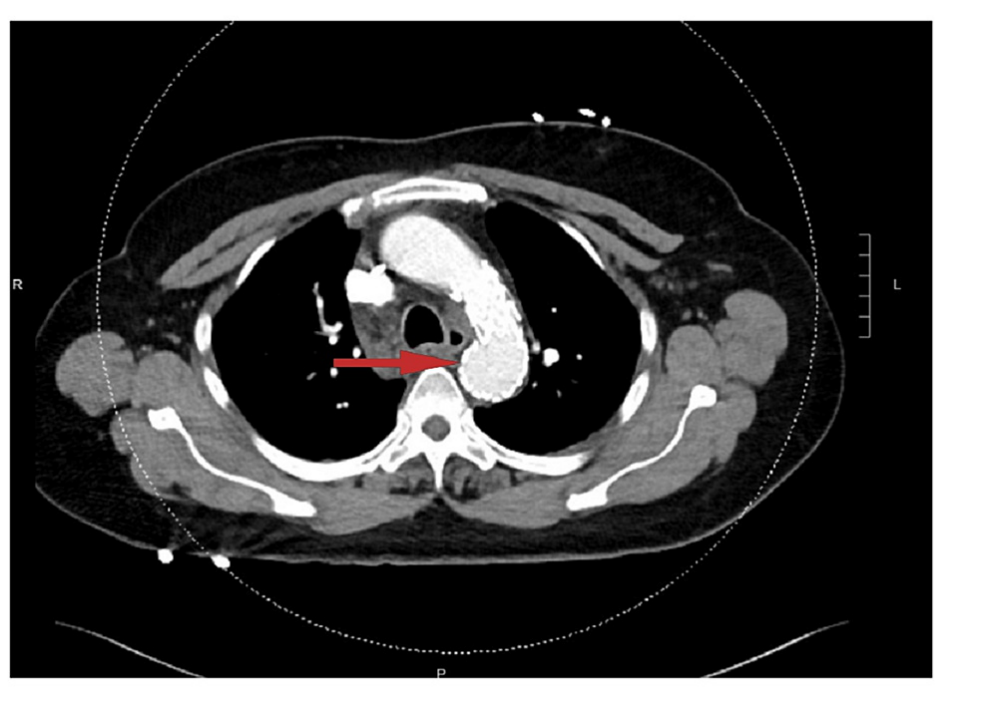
The chest CT angiography image demonstrates the aortic arch subsequent to the successful surgical correction of an aberrant right subclavian artery. The arrow indicates the absence of any residual aberrant right subclavian artery, confirming the effectiveness of the repair.
